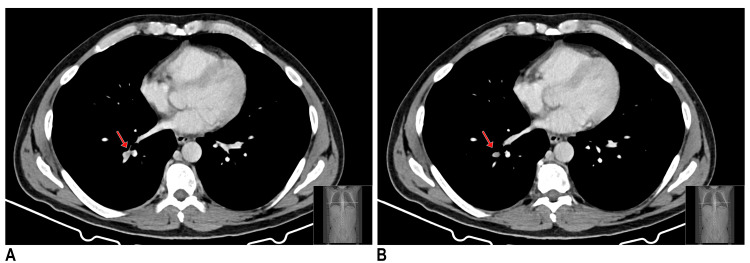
Thoracoabdominal computed tomography axial scan: segmental pulmonary thromboembolism of the right lower lobe of the lung A: segment 1; B: segment 2
